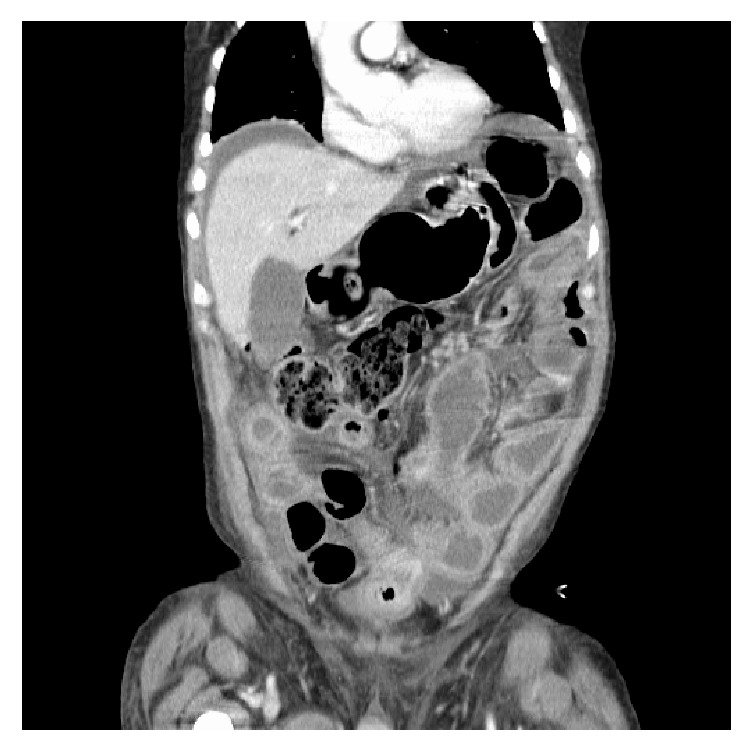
CT abdomen coronal view after IV contrast. There is significant dilatation noted in the loops of the small intestines and some of them showed diffuse wall thickening. There is a large amount of free fluid noted in the abdomen and pelvis. There is free air noted in the abdomen and pelvis indicating bowel perforation. May 2016.
